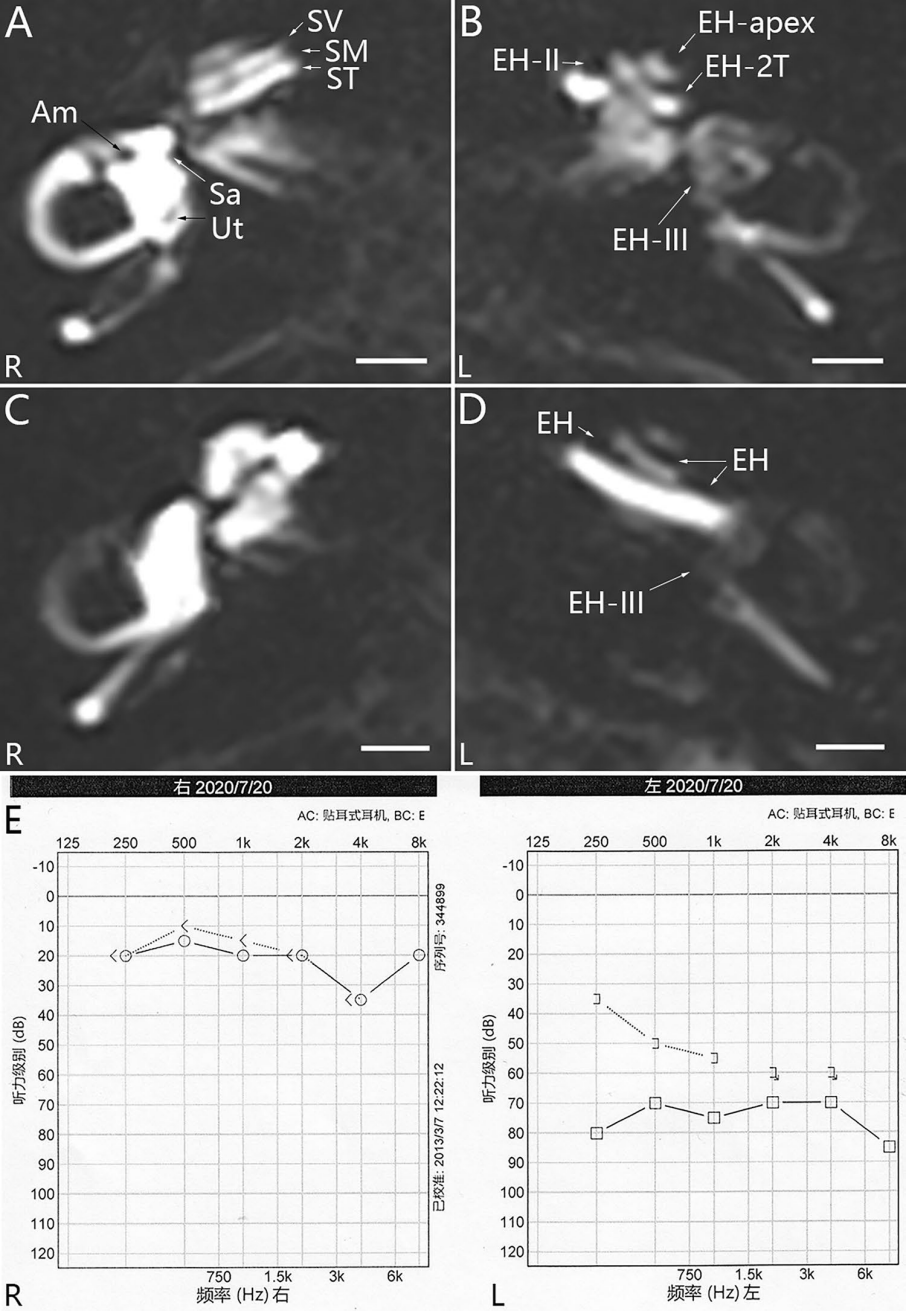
MRI of a patient with unilateral definite Meniere’s disease imaged using hT_2_W–FLAIR–MZFI 24 h after targeted delivery of 20-fold-diluted Gd–DTPA. A 49-year-old male had a history of over 8 years of episodic vertigo lasting for 5 h accompanied by fluctuating right ear hearing loss, tinnitus and aural fullness. The endolymphatic hydrops (EH) levels were grade II in the left (L) cochlea B and grade III in the L vestibule D, but EH was absent in the right ear A, C. EH was obvious in the left apex (EH-apex), which formed an invaginated arc and second turn (EH-2 T) B. There was severe hearing loss and a large air–bone gap at low frequencies in the left ear E. *Am* ampule, *Sa* saccule, *SM* scala media, *ST* scala tympani, *SV* scala vestibule, *Ut* utricle. Scale bars = 3.0 mm
